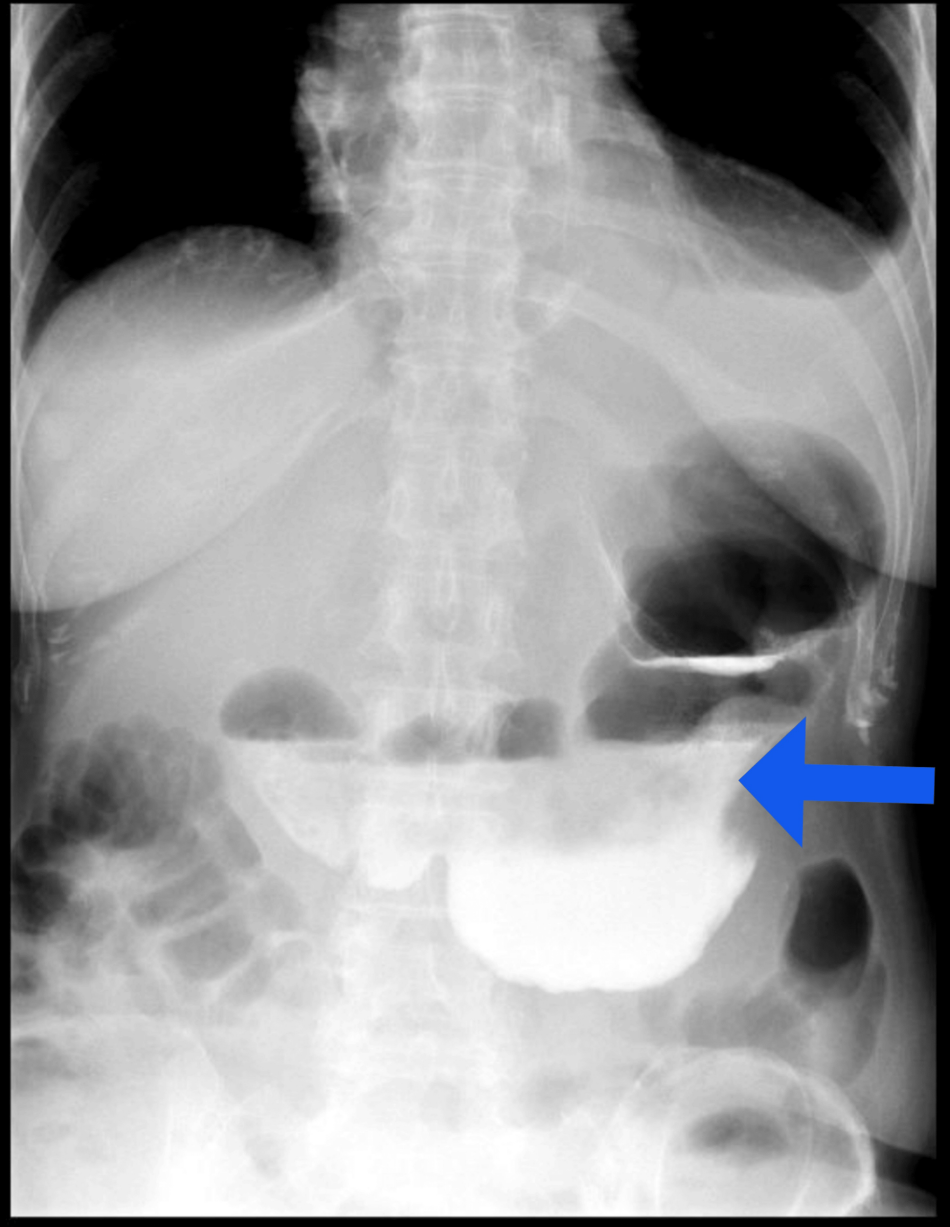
Normal opacified abdominal radiograph confirming adequate postoperative function (blue arrow).
